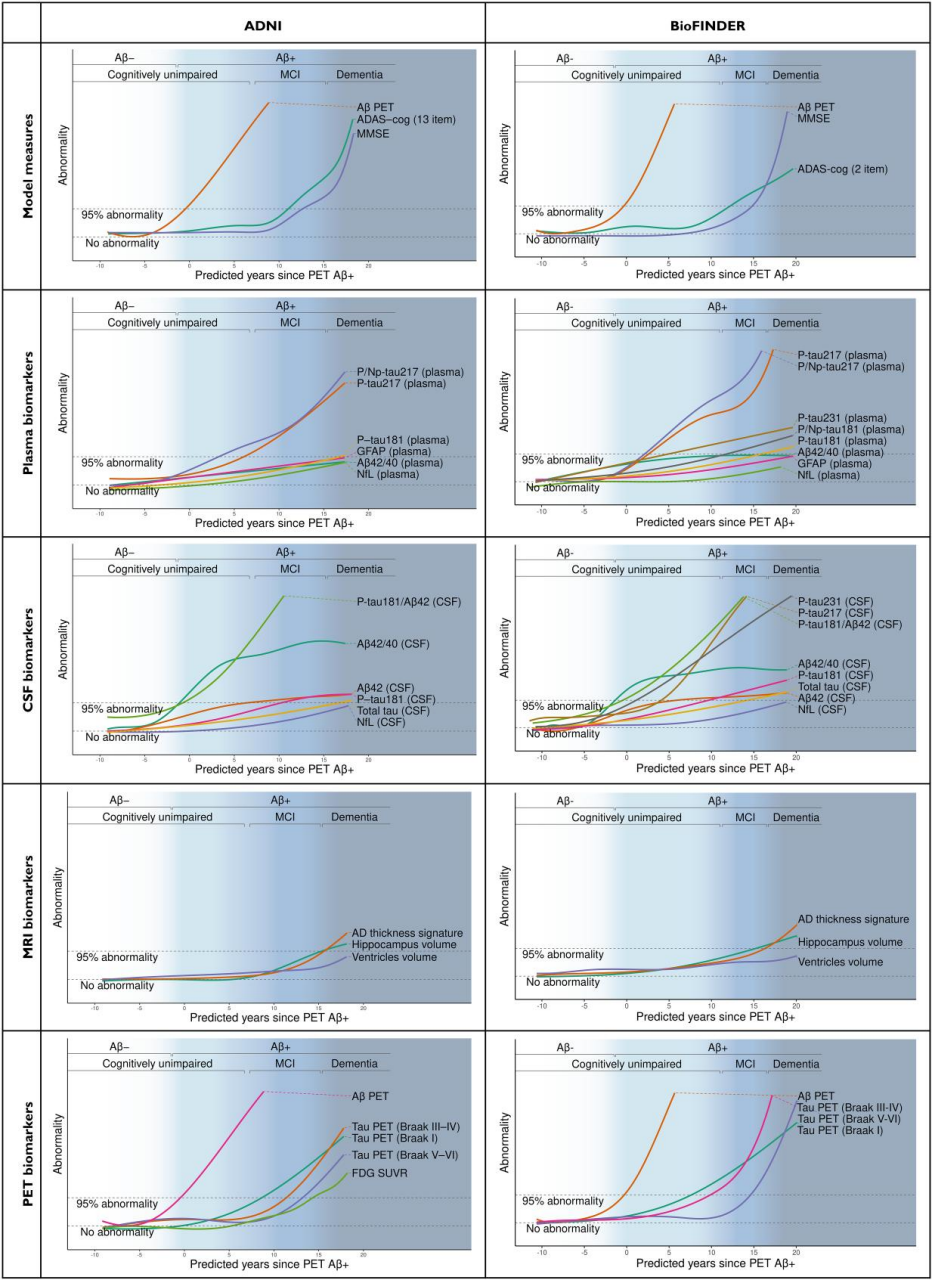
Biomarker trajectories showing abnormality relative to cognitively unimpaired Aβ-negative subjects. Figure shows measures included in the disease progression model, plasma biomarkers, CSF biomarkers, MRI biomarkers and PET biomarkers. Aβ = amyloid-β; ADAS-cog = Alzheimer's Disease Assessment Scale-Cognitive Subscale; MCI = mild cognitive impairment; MMSE = Mini-Mental State Examination; SUVR = standardized uptake value ratio.
